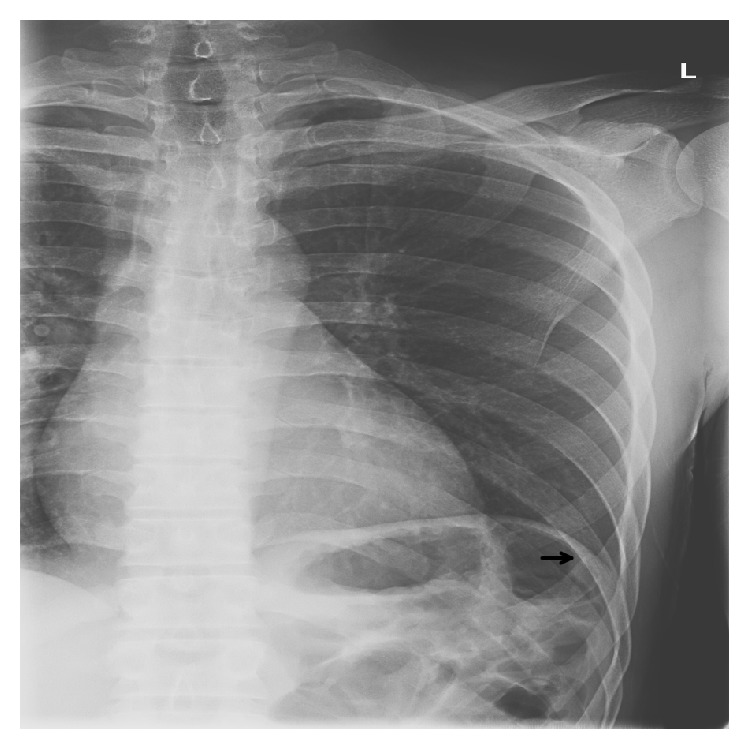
Chest X-ray from the patient's initial ED visit showing anterior lateral left seventh rib fracture (black arrow), with no lung contusion or pleural effusion present.
